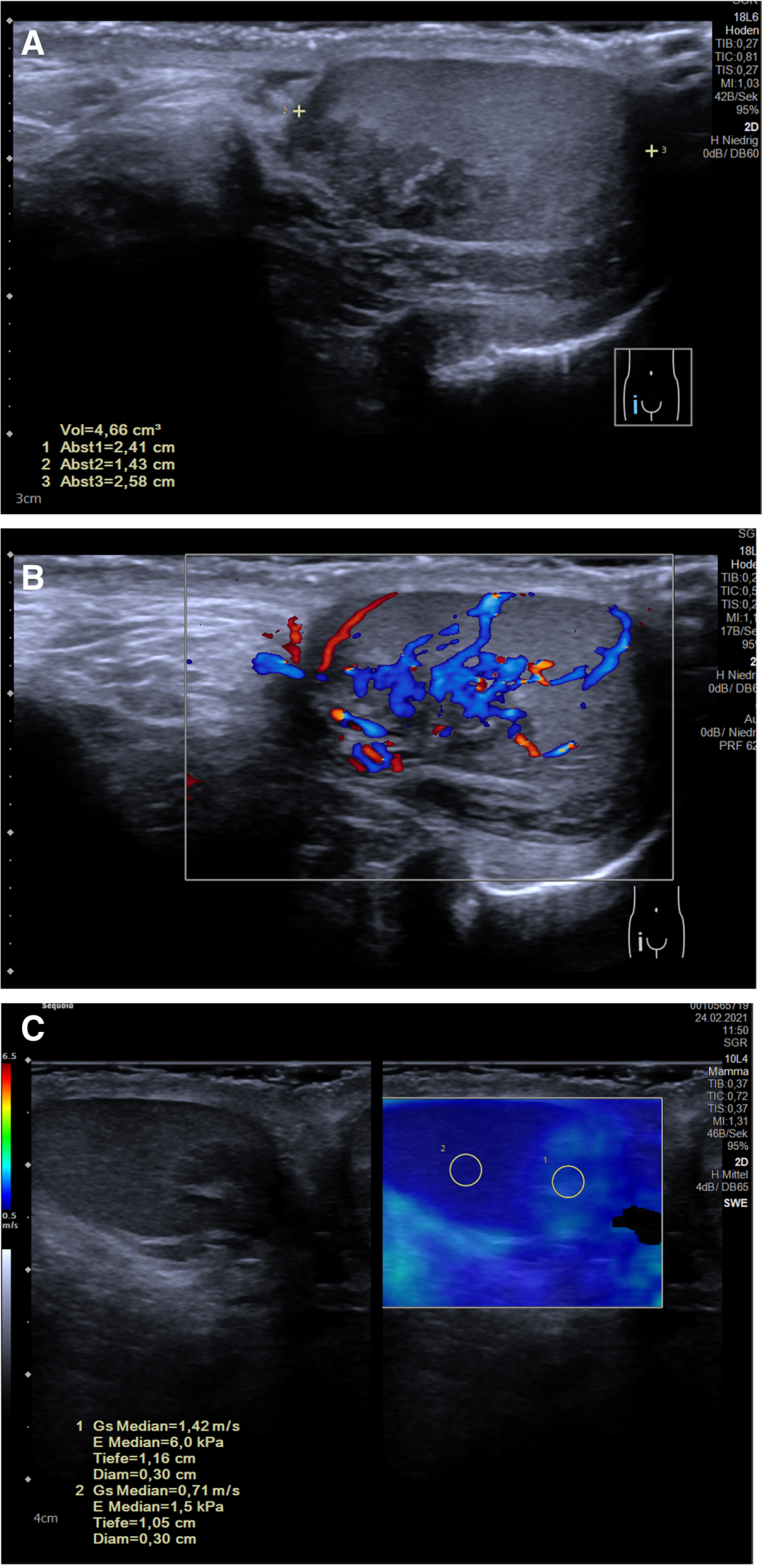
A 13-year-old boy with testicular adrenal rest tumor in adrenogenital syndrome. A B-mode image demonstrating hypoechogenic inhomogeneous lesion in the lateral aspect of the testes (Sequoia, Siemens, L18-5 probe). B Color Doppler mode showed hypervascularization of the tumor (Sequoia, Siemens, L18-5 probe). C SWE demonstrated increased stiffness within the pseudotumor compared to the surrounding physiological tissue (Sequoia, Siemens, L10-4 probe)
